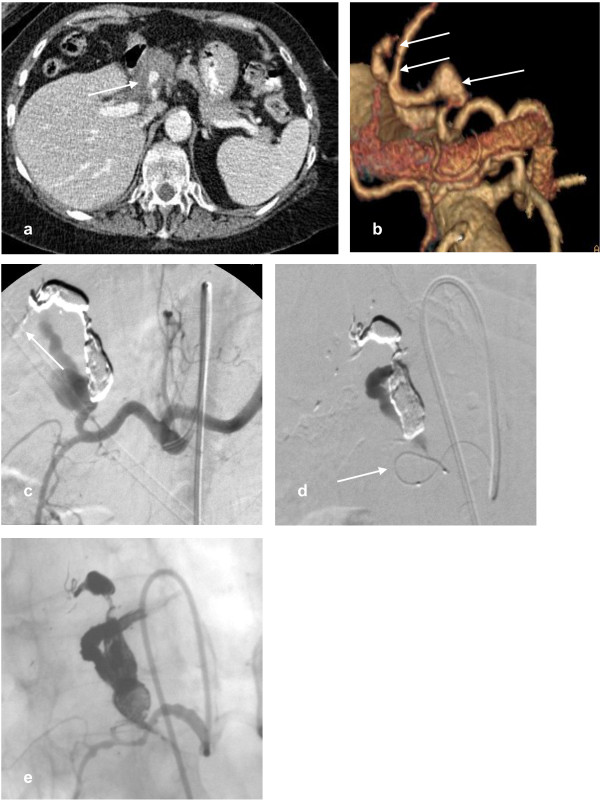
a) A patient with vasculitic hepatic artery aneurysms presented following minor trauma. Axial contrast enhanced CT demonstrates haematoma around a pseudoaneurysm (arrow) indicating that this is the likely cause of recent haemodynamic instability. b) 3D volume rendered reconstruction demonstrates 3 aneurysms arising from a branch of the left hepatic artery (arrows). The right hepatic artery arose from the SMA. c) Selective arteriogram of the coeliac axis with standard catheter after 2 aneurysms had been embolised with onyx (ev3, Irvine, CA, USA). The cast of the onyx is demonstrated, and some distal embolisation (arrow) of onyx. d) A microcatheter is demonstrated within the final bleeding aneurysm (arrow). e) A selective angiogram demonstrates onyx filling all aneurysms and maintained patency of the gastroduodenal artery.
